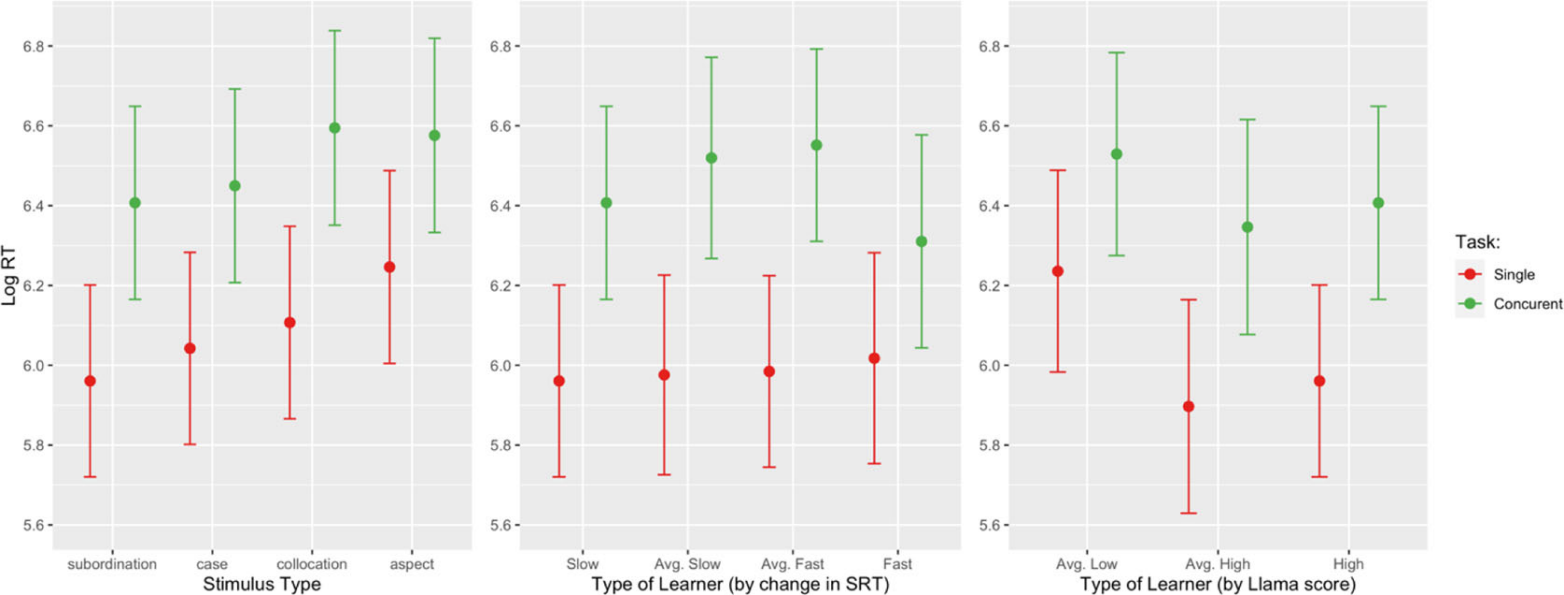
Response latencies for the four stimulus Types across ST and CT conditions (left panel); response latencies for the four types of implicit learners across both Conditions (middle panel); response latencies for the three types of explicit learners across Conditions (right panel). Whiskers represent the 95% lower and upper confidence interval limits.
